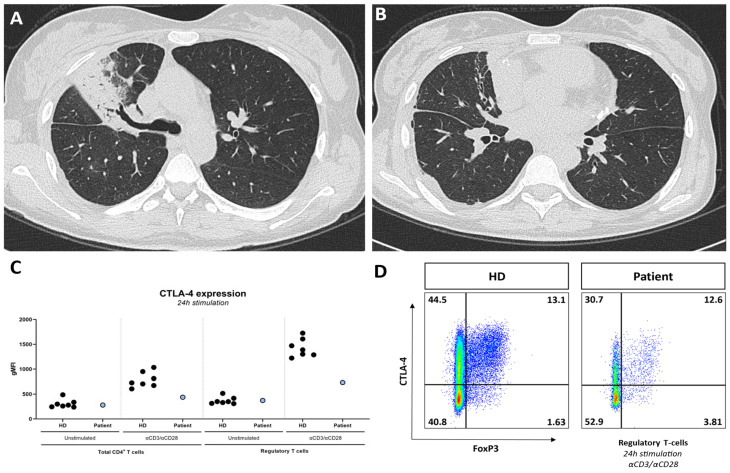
CT-scan showing lobar pneumonia (A) and focal bronchiectasis (B); (C) CTLA-4 expression on CD4^+^ T-cells and regulatory T-cells, unstimulated and after 24 h stimulation with aCD3/aCD28, in the patient and healthy donors (HDs), showing decreased CTLA-4 expression on CD4^+^ T-cells and regulatory T-cells during stimulated conditions in the patient compared with HDs; (D) decreased CTLA-4 expression FoxP3^+^ regulatory T-cells demonstrated through flow cytometry.
